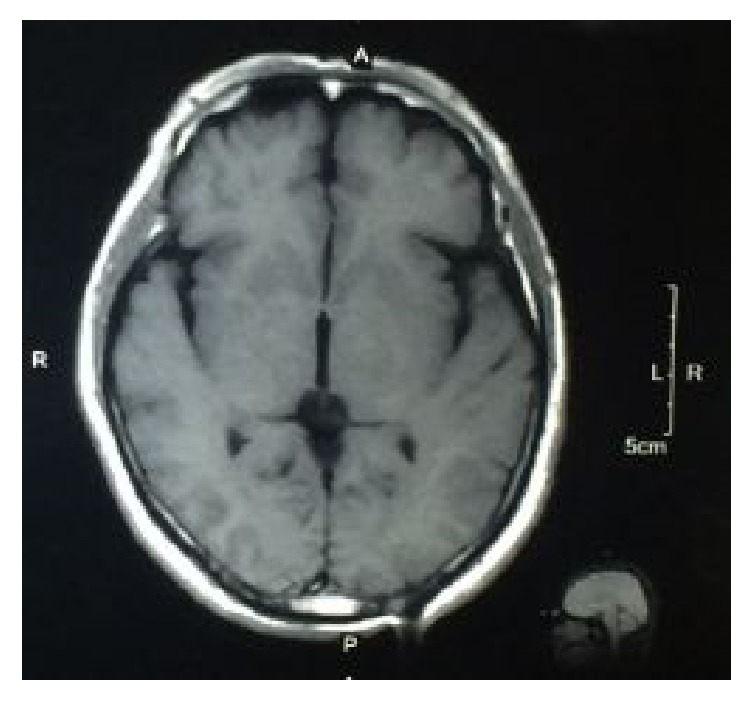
Magnetic resonance imaging of the brain; axial section of the brain is shown. The image shows normal study of the brain.
